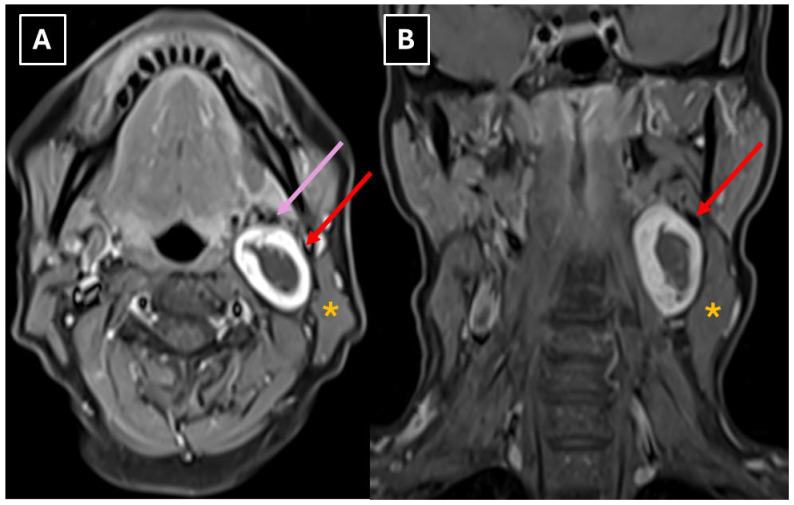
Post-contrast MRI scan of the neck. (A): Axial T1-weighted TSE Dixon (water-only) and (B) coronal T1-weighted TSE Dixon (water-only) show predominantly low-to-intermediate signal intensity with a thick, smoothly marginated peripheral rim of avid contrast enhancement and a central non-enhancing, hypovascular hypointense area—a destructive cavity, consistent with cystic degenerative components. Red arrow—internal carotid artery; pink arrow—external carotid artery; orange asterisk—sternocleidomastoid muscle.
